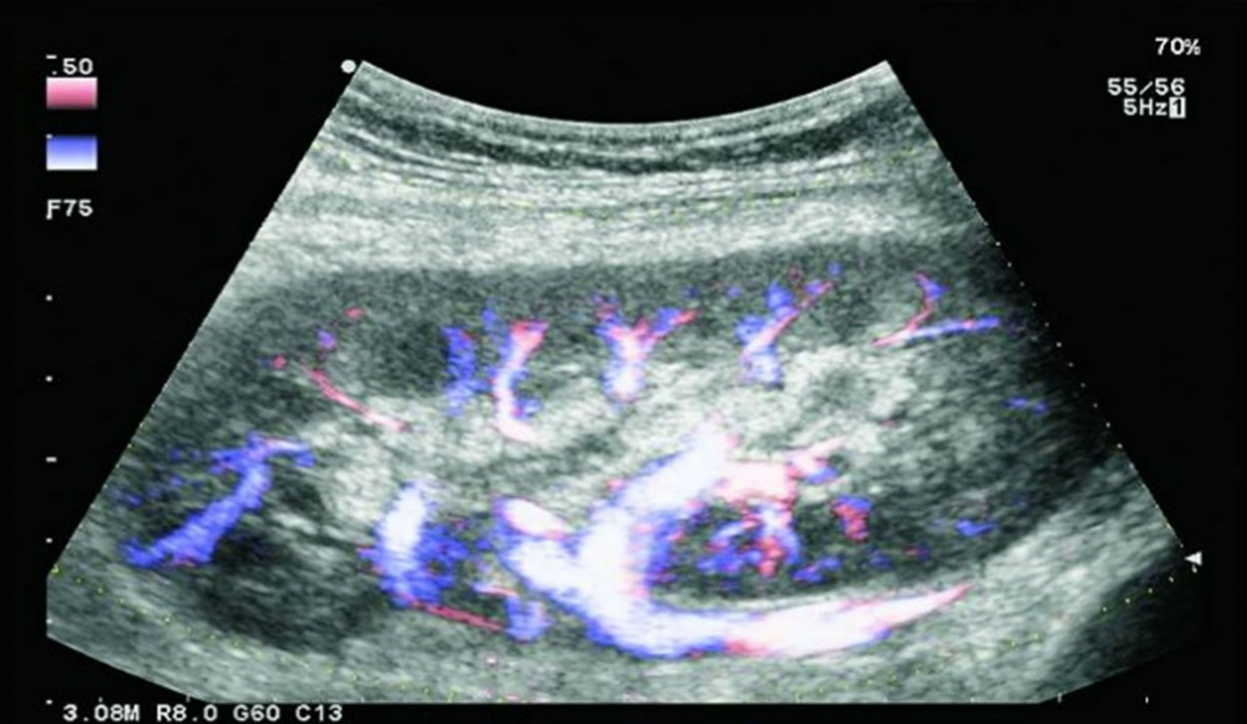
Renal ultrasound five years after transplantation.
